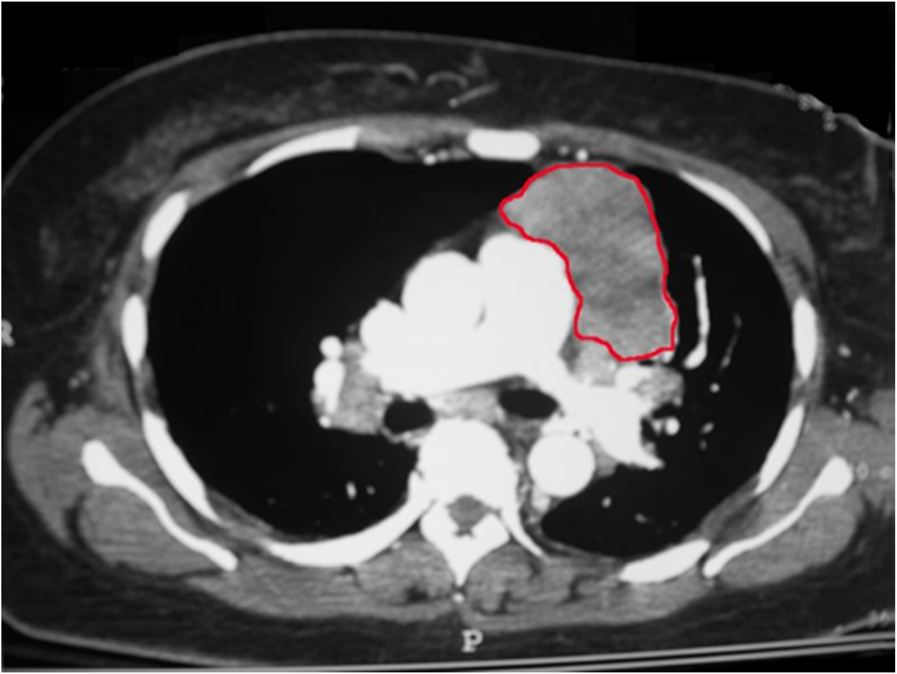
Chest CT showing a large mediastinal mass outlined in *red* and found subsequently to be a thymoma
